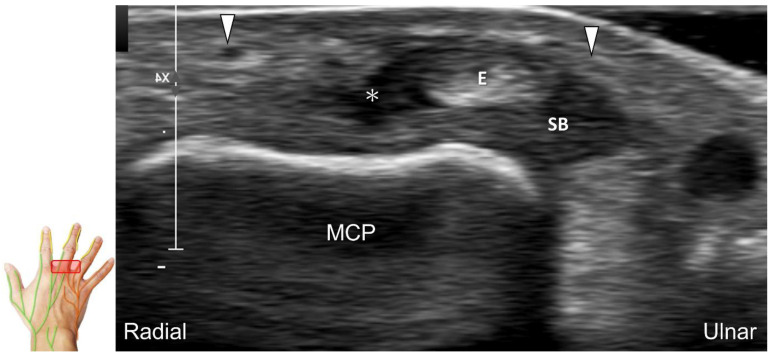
Sonographic imaging reveals irritation of the ulnar aspect of the dorsal common digital nerve due to extensor tendon subluxation resulting from a tear in the sagittal band. Arrowheads: dorsal common digital nerve; asterisk: tear of the sagittal band. SB: sagittal band; E: extensor tendon; MCP: metacarpal bone.
